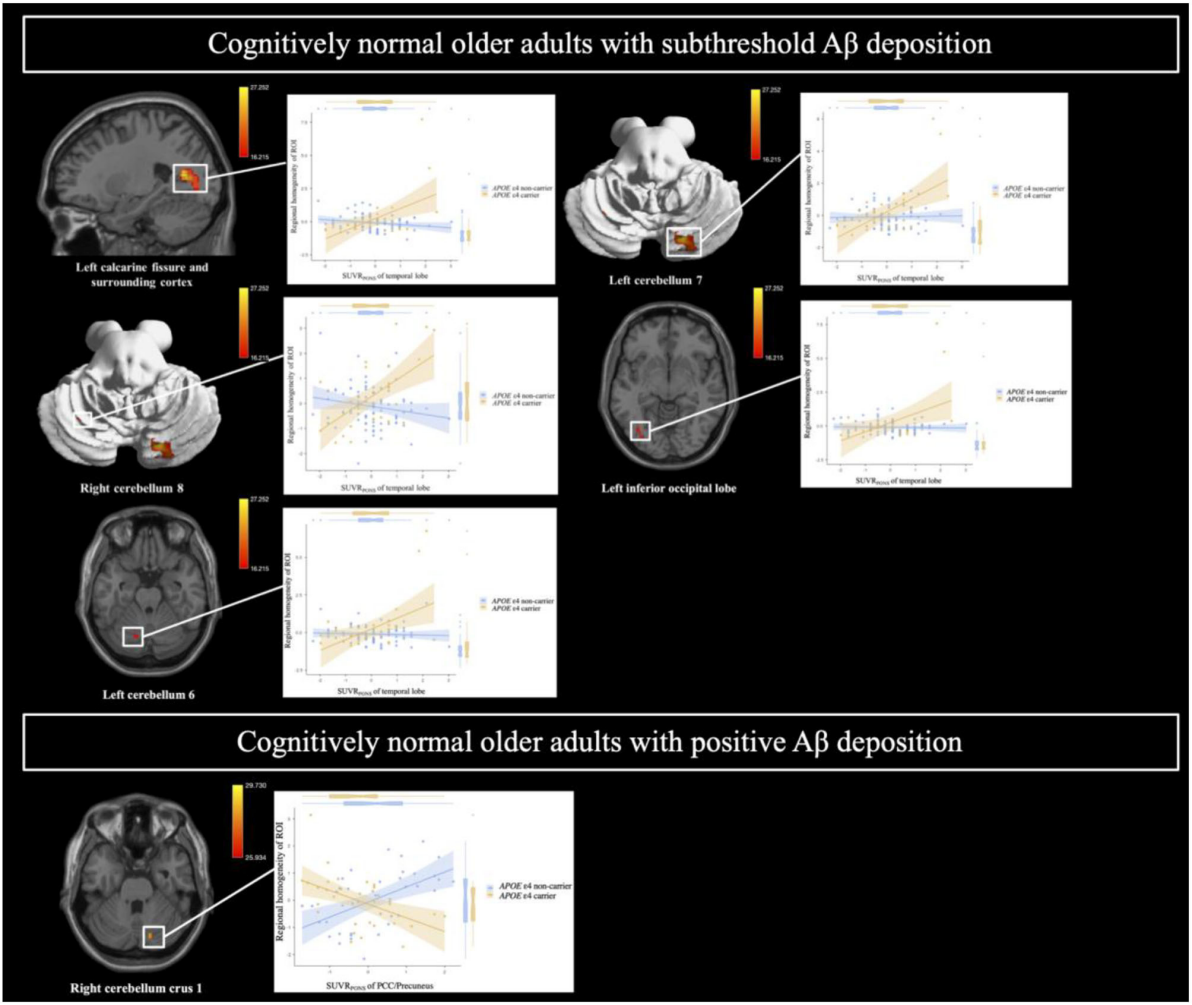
Brain regions showing a significant interaction between Aβ deposition and *APOE* ε4 carrier status for regional homogeneity in cognitively normal older adults with subthreshold and positive Aβ deposition. General linear model (GLM) analysis adjusted for age, sex, and years of education (FDR-adjusted *P* < 0.05). Each variable was z-transformed using the mean and standard deviation. SUVR_PONS_, standardized uptake value ratios of [^18^F] flutemetamol (FMM).
